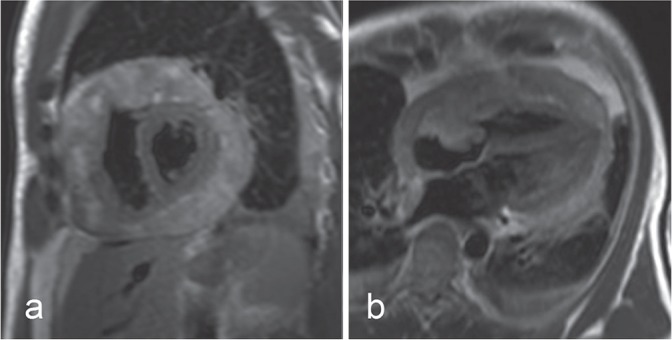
T_2_-weighted “dark blood” turbo spin echo images in (a) short axis and (b) four-chamber plane confirm echocardiographic findings of tumorous mass protruding into the right atrium and soft tissue filling the pericardial sac around both ventricles.
